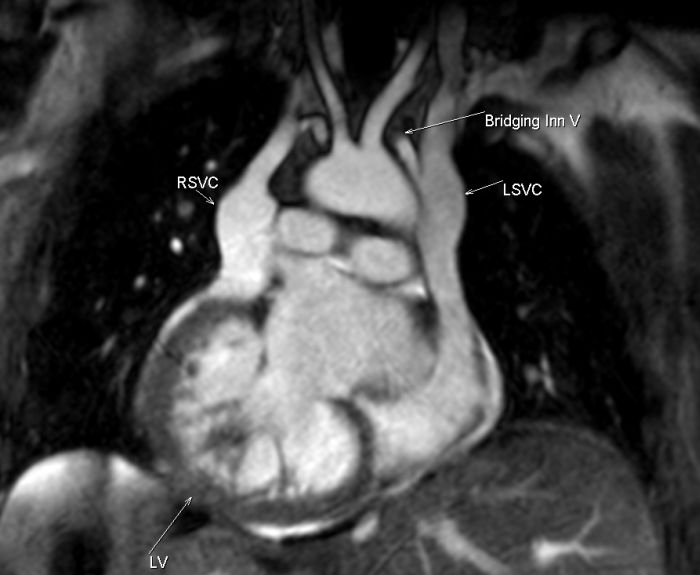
Coronal Cardiac MRI image of the patient showing the presence of the right persistent superior vena, left superior vena cava and bridging inominate vein.
